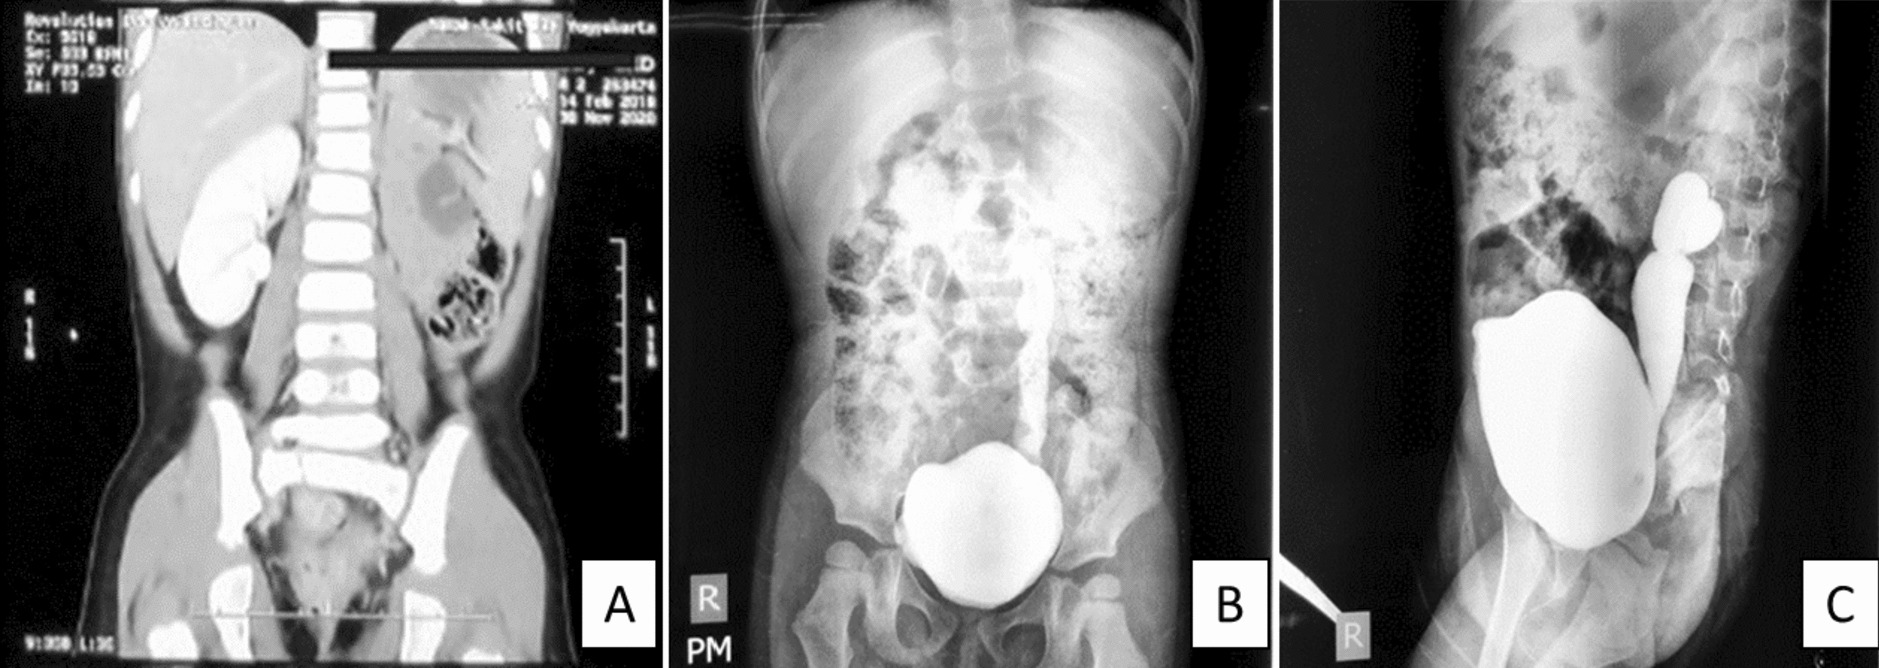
Radiological examination A. Abdominal CT-scan with contrast showed the absence of left kidney. B, C Voiding Cystourethrography revealed gross dilatation and ureteral tortuosity of left ureter (vesicoureteral reflux grade 5), without observed dilatation of right ureter (grade 1)
